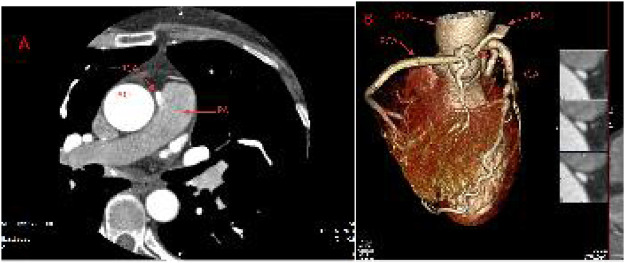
Preoperative coronary CTA. (A) Abnormal origin of the RCA from the pulmonary trunk (red arrow); (B) VR-reconstructed three-dimensional image shows the abnormal origin of the RCA (red arrow).
